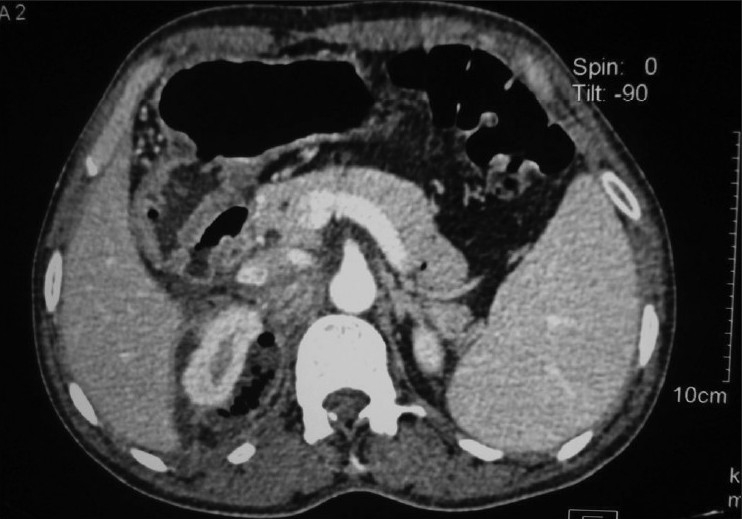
Axial CT image through the upper pole of right kidney showing perinephric abscess reaching posterior to IVC
